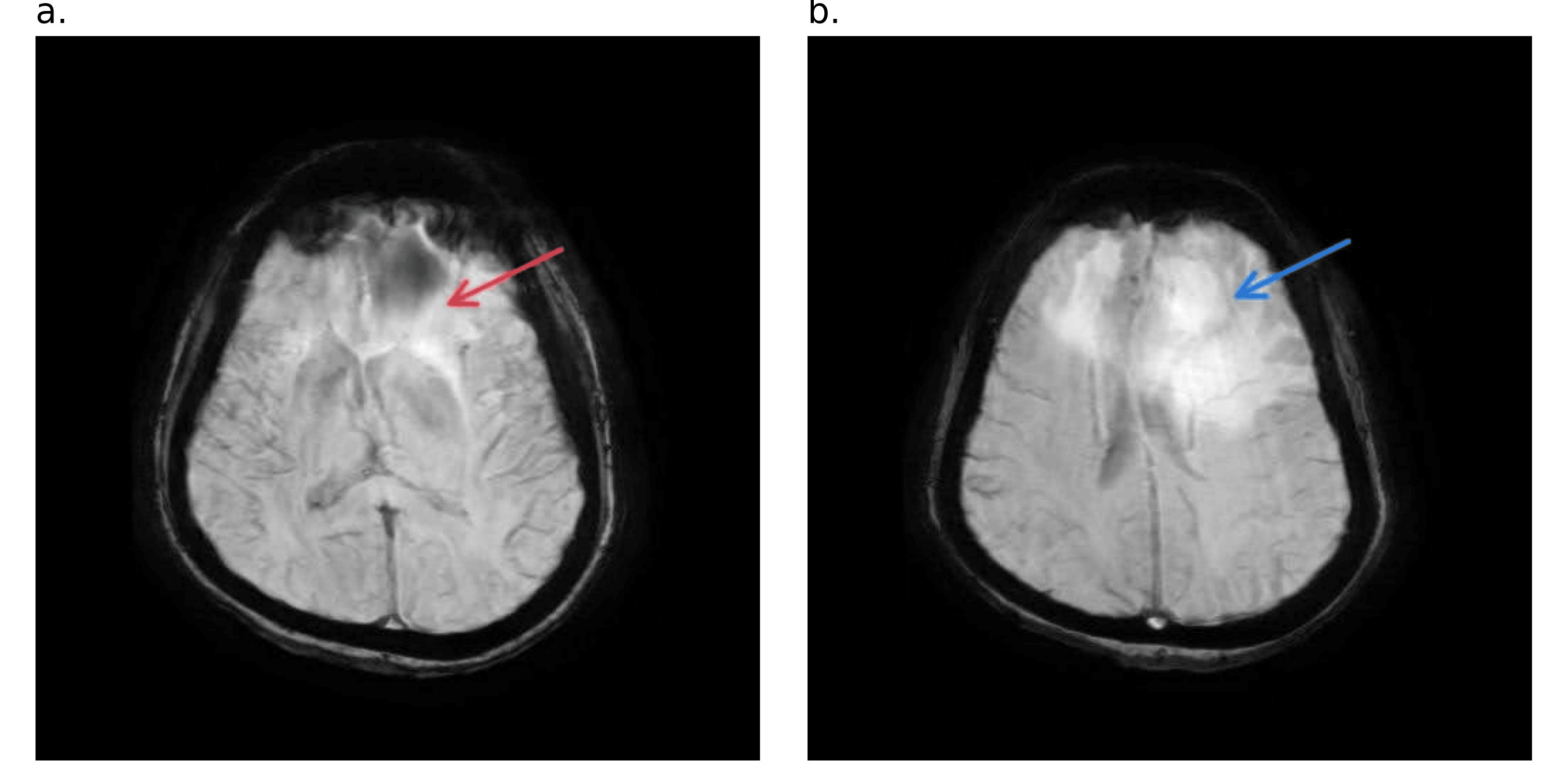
Axial SWI MRI image a. At the level of the capsuloganglionic region. b. At the level of the body of the lateral ventricle MRI: magnetic resonance imaging; SWI: susceptibility weighted imaging
